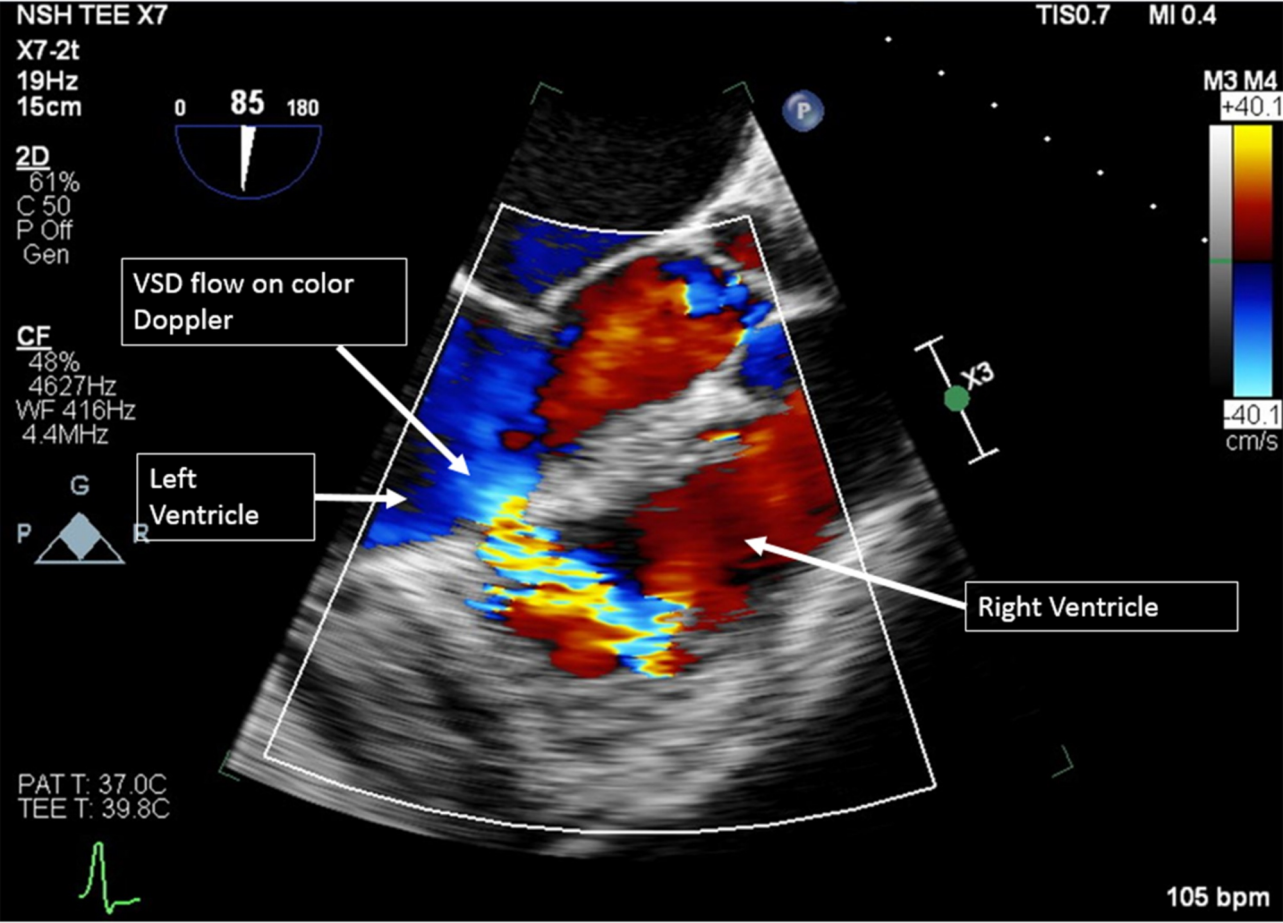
Transthoracic echocardiogram (TTE) showing VSD flow VSD: ventricular septal defect
